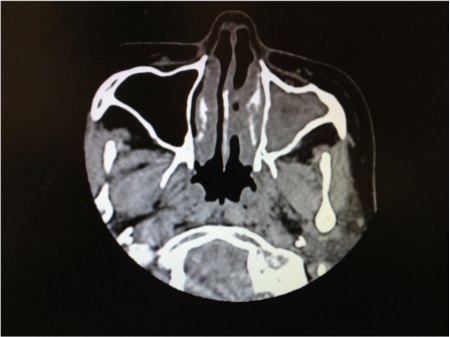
Chest computed tomography scan showing left ethmoidal and maxillary sinusitis
